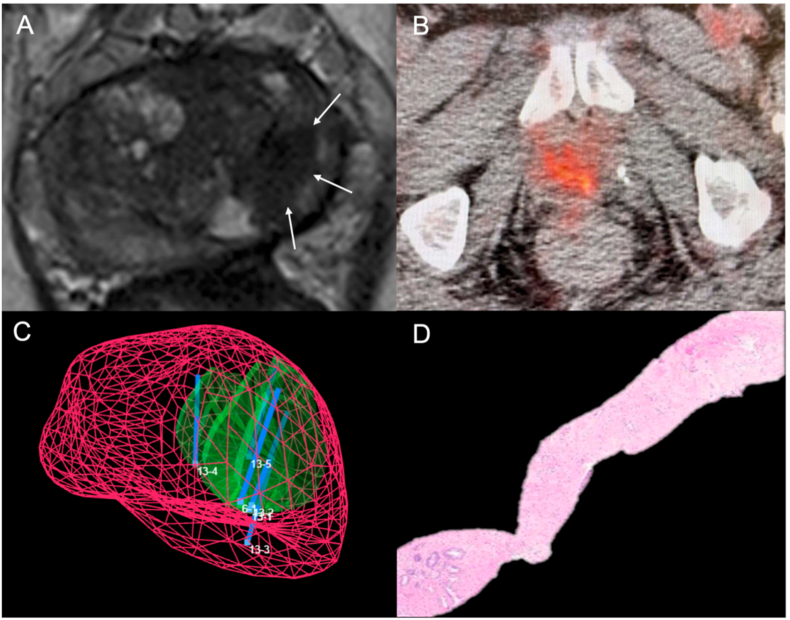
Follow-up MRI in October 2022 showed treatment effect and no suspicious regions (Fig. 3A). PSMA scan at that time showed disappearance of the PSMA hotspot (Fig. 3B). Biopsy targeting the site of the previous PSMA hotspot and adjacent left lobe (Fig. 3C) revealed only fibrosis upon pathologic analysis (Fig. 3D). One year post-treatment PSA is 0.7 ng/ml, and urinary and sexual function are unchanged from pre-treatment status (using alfuzosin and sildenafil as before).
